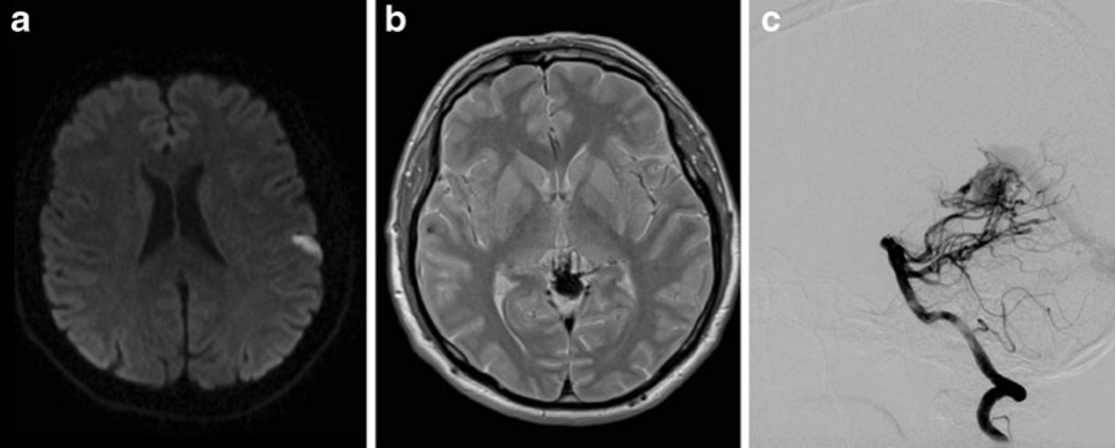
A 55-year-old male with sudden onset of transitory aphasia. a DWI (b-factor 1000) shows an area of restricted diffusion in the left parietal region. b PD-weighted SE image shows an AVM in the pineal region. c DSA proves a combined pial-dural AVM. Although the precise pathogenesis of acute neurological symptomatology in a patient with an AVM is unknown, the “steal phenomenon” may play a role in its development. DWI, diffusion-weighted imaging; PD, proton density; SE, spin echo; AVM, arteriovenous malformation; DSA, digital subtraction angiography
